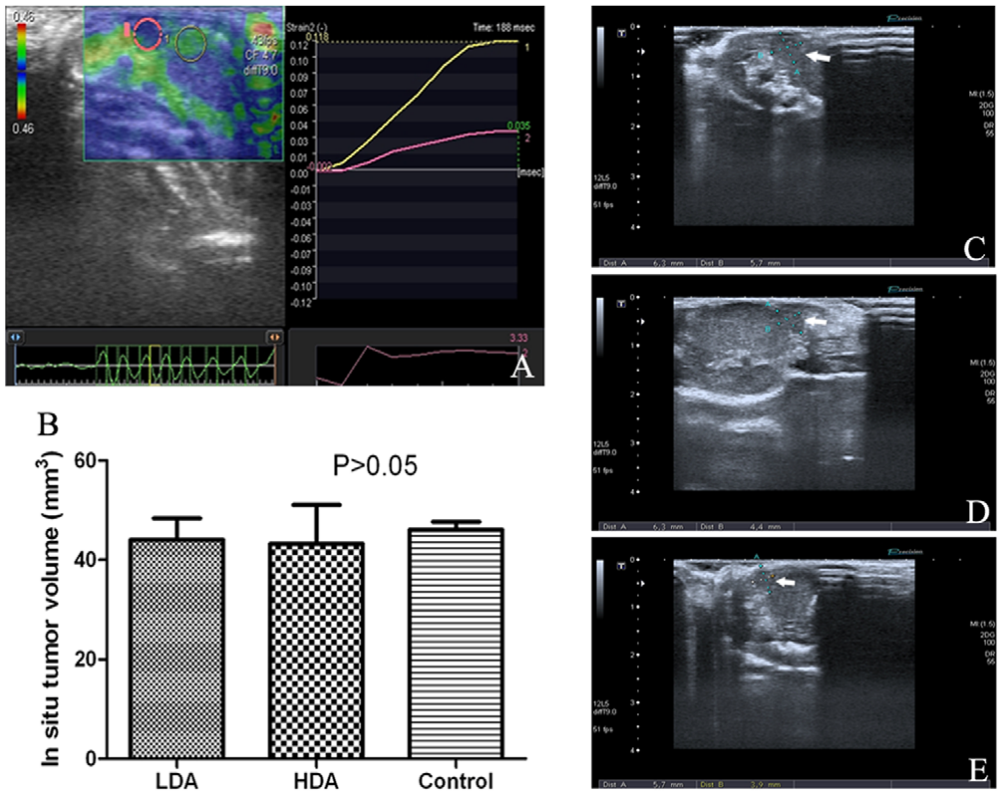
Representative images of ultrasonography. B ultrasound scan with ultrasonic elasto-test was carried out 4 weeks after the in situ implantation. The elasticity of the low-echo hepatic region (within the pink circle) was much lower than that of the normal-echo hepatic region (within the yellow circle), as shown in picture A. Thus, it could be deduced that the low-echo region should be the in situ implanted malignancy. Picture C, D and E are representative ultrasound images of LDA group, HDA group and control group, respectively. Figure B shows there was no statistical difference in mean tumor volume among each group (44.03±7.51 mm^3^, 43.24±13.55 mm^3^, and 46.07±4.57 mm^3^, respectively, P>0.05).
